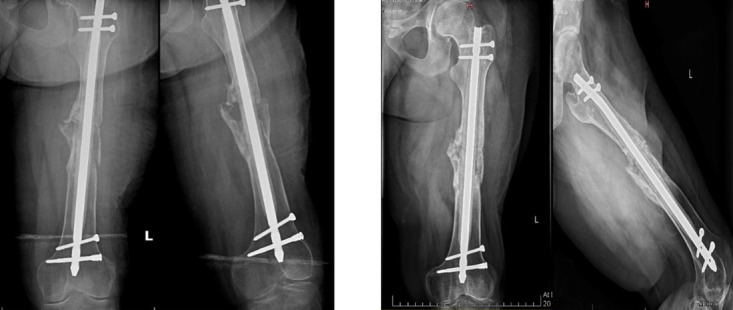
Immediate postoperative (left) and six-month follow-up radiographs (right) of the left femur demonstrating stabilization with an antibiotic-coated intramedullary SIGN nail and progressive radiological union.
